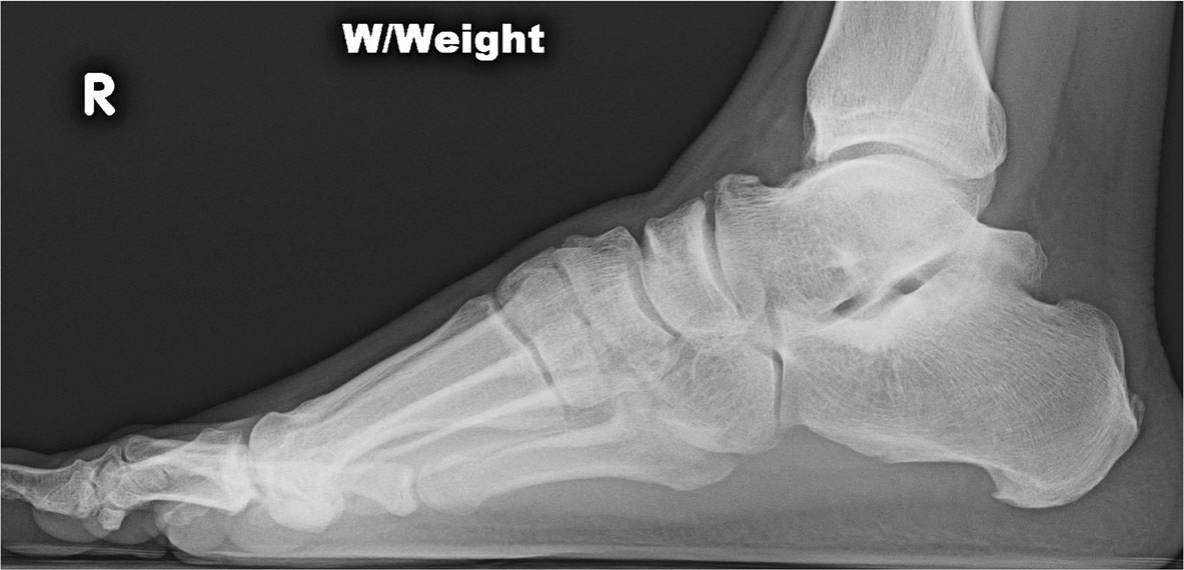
A lateral radiograph demonstrates an elongated posterolateral (trigonal) process of the talus (Stieda process)
